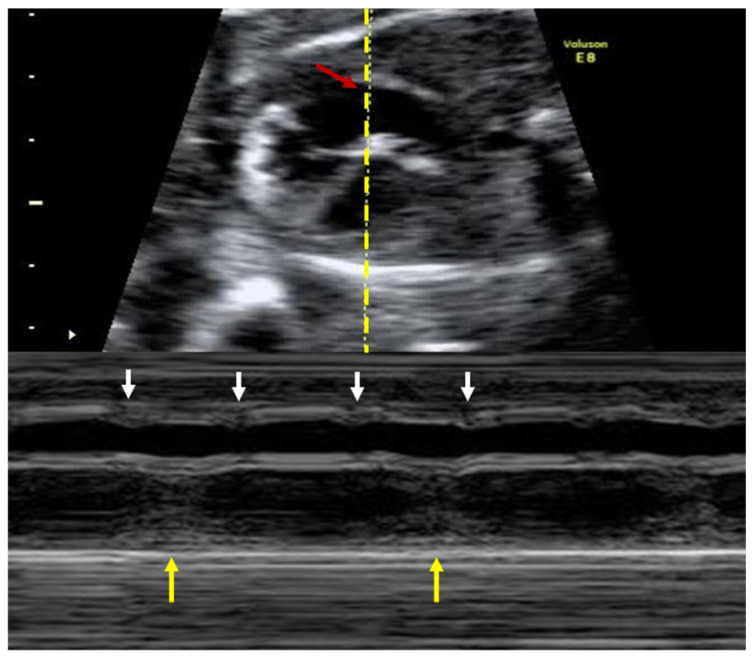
M-mode echocardiography used to demonstrate the fetal third-degree atrioventricular block. The atrial and ventricular activities are completely dissociated. Red arrow, dotted yellow line: section plane. Small white arrows: ventricular contractions. Large yellow arrows: atrial contractions. Courtesy of Anca Panaitescu, Filantropia Clinical Hospital, Bucharest.
